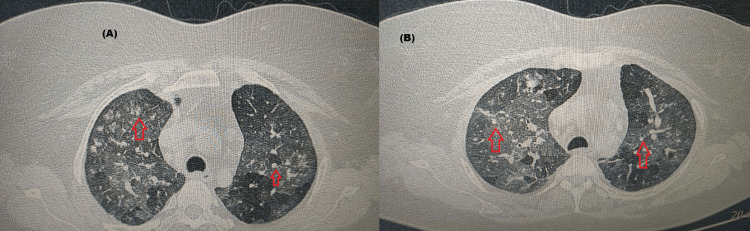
High-resolution CT (HRCT) chest images of the patient at two different CT cuts Figure (A): Multiple centrilobular nodular opacities (red arrow) coalescing to form patches of consolidation Figure (B): Multiple centrilobular nodular opacities (red arrow) Both (A) and (B): mediastinal and hilar lymphadenopathy and multifocal discrete patches of ground glass opacities with intervening areas of normal lung parenchyma giving mosaic attenuation appearance
